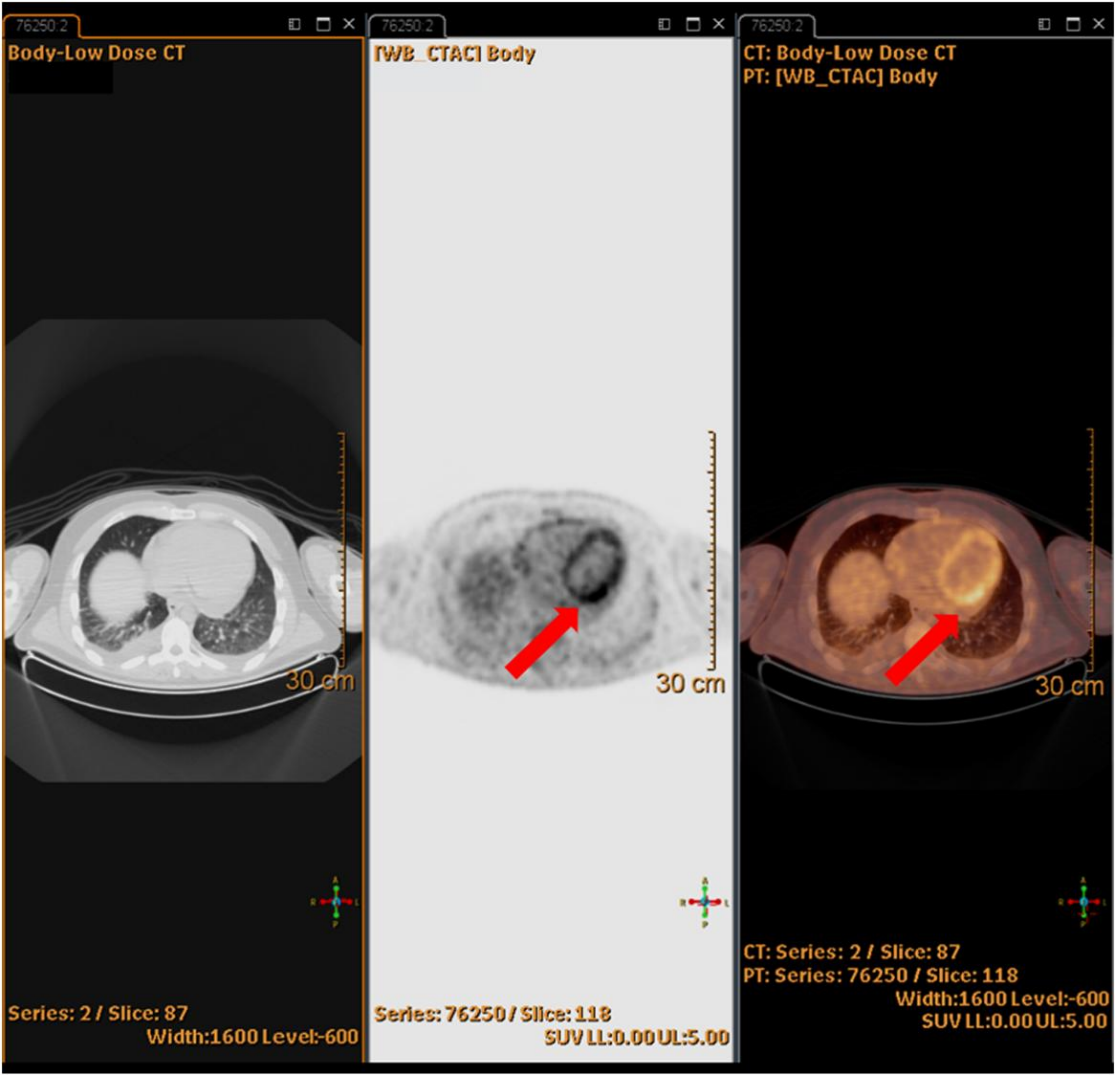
On the left, low-dose body CT scan; in the middle, grey-scale PET-FDG highlighting intense glucose uptake and metabolism in the pericardium as compared to the hepatic dome; on the right, low-dose CT–PET-FDG fused image.
